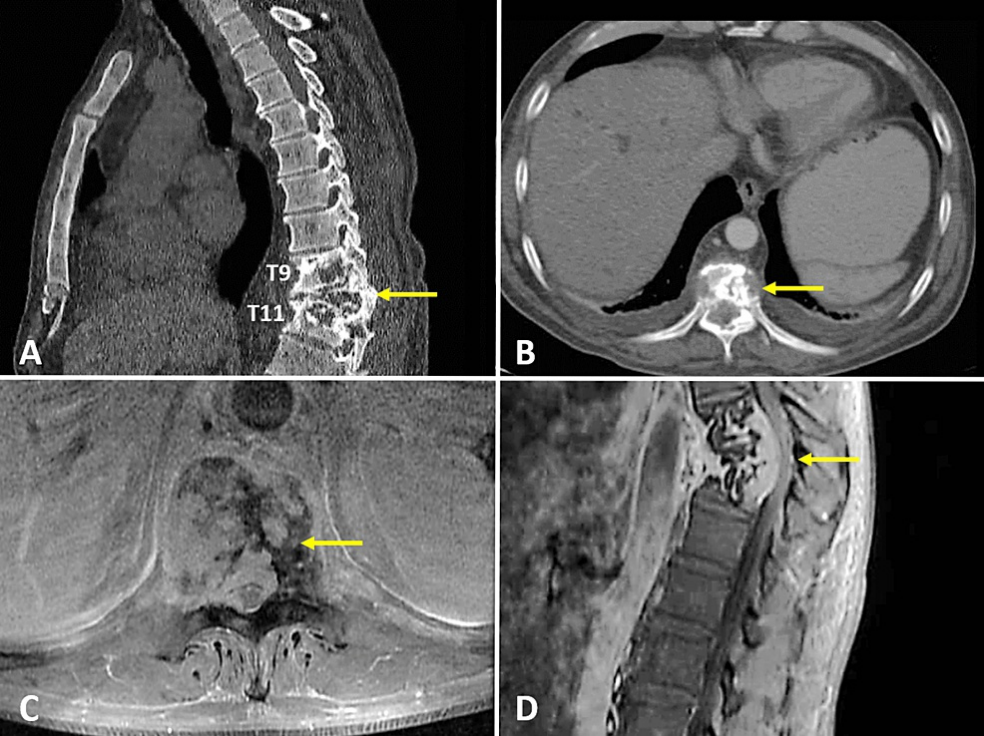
Preoperative imaging studies (A) Sagittal CT section with osteolytic lesions at the levels of T9, T10, and T11, with the presence of almost complete vertebral collapse of T10 and increased thoracic kyphosis (yellow arrow); (B) CT axial section at T10 level with osteolytic lesions (yellow arrow), which partially invade the spinal canal and the posterior column of the vertebra; (C) Axial contrast-enhanced MRI at the T10 level with the presence of a heterogeneously contrast-enhancing tumor lesion with total occupation of the vertebral body and invasion of the vertebral canal (yellow arrow); (D) Sagittal contrast-enhanced MRI with a tumor lesion with anterior and posterior components to the vertebral bodies of T9, T10, and T11 with compression of the spinal cord (yellow arrow).
